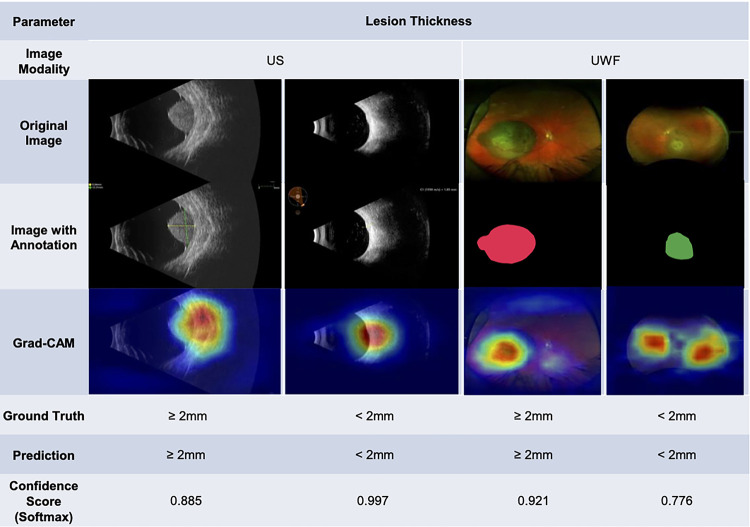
Representative ultrasound (US) and ultra-widefield fundus (UWF) original images, with a Grad-CAM saliency map showing the areas of highest probability for the thickness prediction model.
